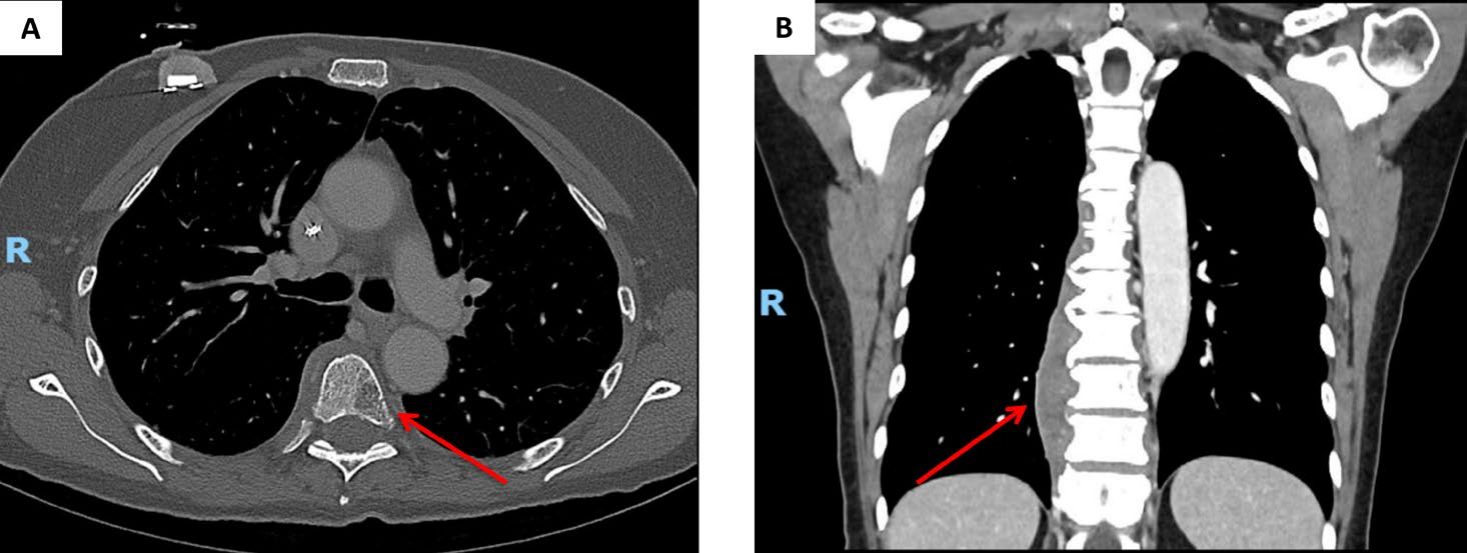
(A) Axial plane computed tomography scan of the chest demonstrating a 1.7 × 1.2‐cm lytic focus within the T6 vertebral body (red arrow). (B) Coronal plane computed tomography scan of the chest showing a right‐sided paravertebral mass measuring 13.6 cm in the craniocaudal dimension spanning from the T5‐6 level to T12. CT‐guided FNA of this mass would later confirm involvement of the patient's CLL/SLL consistent with recurrence.
